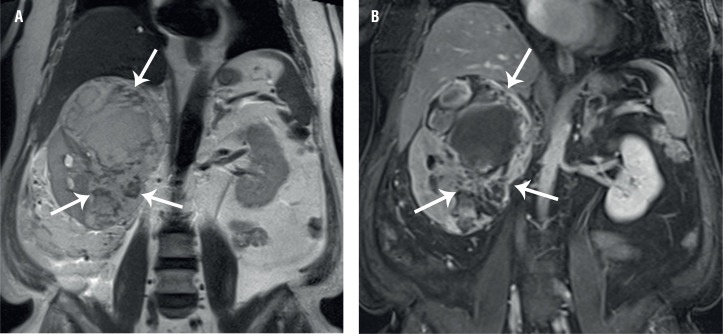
Coronal T2-weighted (A) and fat-suppressed contrast-enhanced T1-weighted (B) MR images demonstrating a tangled mesh of signal-void tumor vessels (arrows in A) in the periphery of the mass, corresponding to the areas with strong enhancement (arrows in B).
